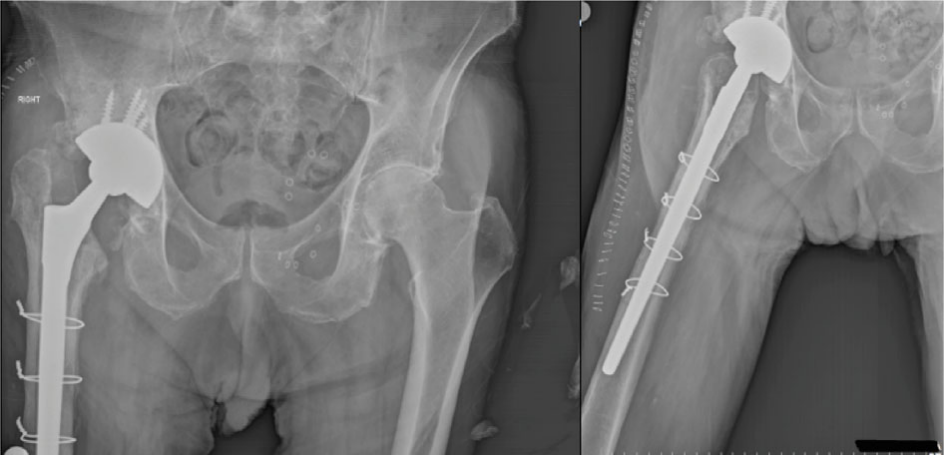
Postoperative anteroposterior and lateral hip x‐rays after the second stage (reimplantation) with a cementless grit‐blasted titanium femoral stem and a porous‐tantalum acetabular cup; radiographs obtained 6 weeks after reimplantation.
